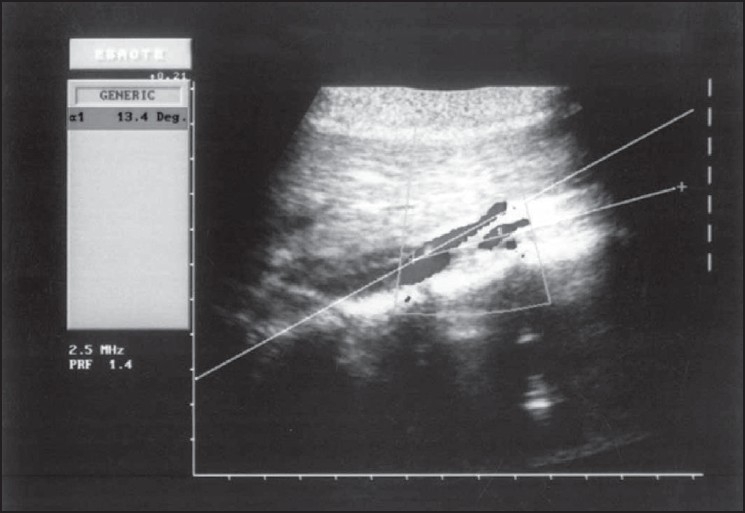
Abdominal Doppler ultrasound demonstrating aortomesentric angle of 13 degrees
